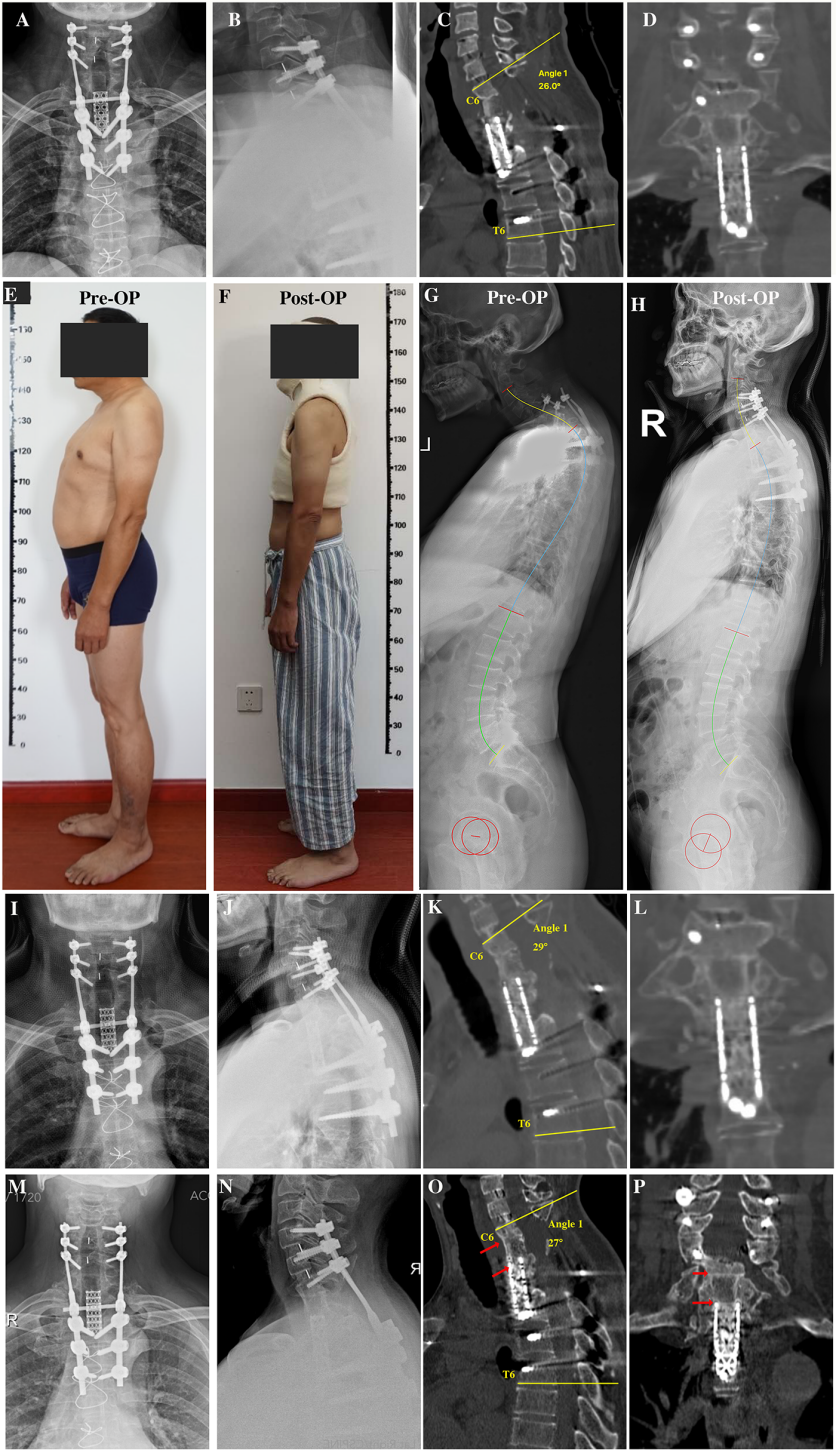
(A,B) Anteroposterior and lateral x-rays after revision surgery. (C,D) Sagittal and coronal reconstruction of CT following revision surgery showing recovery of local kyphosis. (E) Preoperative lateral photographs of the patient. (F) Lateral photograph of the patient wearing a cast postoperatively. (G) Preoperative full-length lateral spine radiograph of the patient. (H) Postoperative whole spine lateral radiograph of the patient. (I,J) Anteroposterior and lateral x-rays 6 months after revision surgery before removing head-neck-chest cast. (K,L) Sagittal and coronal CT reconstruction 6 months after revision surgery showing bone fusion between the iliac bone and mesh cage graft. (M,N) Anteroposterior and lateral x-rays 3 years after revision surgery showing no instrumentation failure. (O,P) Sagittal and coronal reconstruction of CT 3 years after revision surgery showing bone fusion among the C6 inferior endplate, iliac bone, and mesh cage graft. CT, computed tomography.
